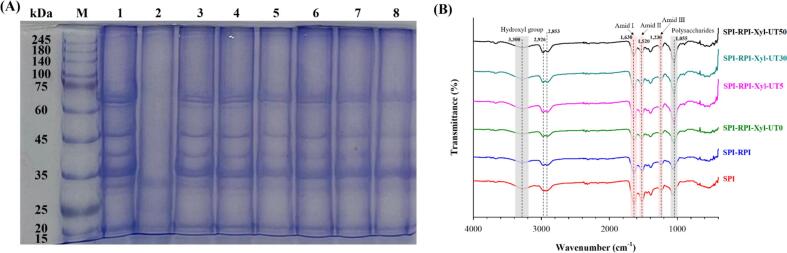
SDS-PAGE profiles (A) and FT–IR spectrum (B) of cooked artificial muscles with varying contents of soy protein isolate (SPI), rice protein isolate (RPI), and D-xylose (Xyl), and different ultrasonic times (UT). M: standard protein marker; line 1: raw SPI; line 2: raw RPI, line 3–8: artificial muscle samples (SPI, SPI-RPI, SPI-RPI-Xyl-UT0, SPI-RPI-Xyl-UT5, SPI-RPI-Xyl-UT30, SPI-RPI-Xyl-UT50).
